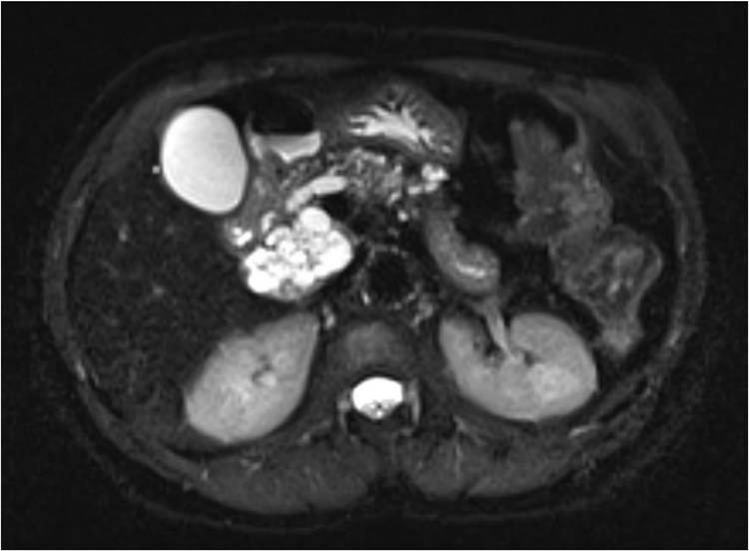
Increased diameter of lesion and dilation of main pancreatic duct on interval MRI.
